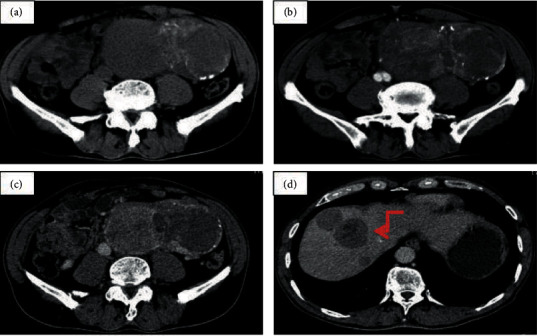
One case of a 65-year-old female that had a slightly higher-level CA199, CA125. (a) Noncontrast CT scan showed an irregular tumour size of about 140 × 81 mm with heterogeneous low density in the retroperitoneal. The lesion presence multiple punctate strip calcifications. (b, c) Contrast-enhanced CT scans depicted heterogeneous nonsignificant enhancement. The CT values of the noncontrast, arterial, and venous phases were 43, 67, and 65 HU. The postoperative CT diagnosis was paraganglioma and (d) liver multiple metastasis (arrow) occurred.
